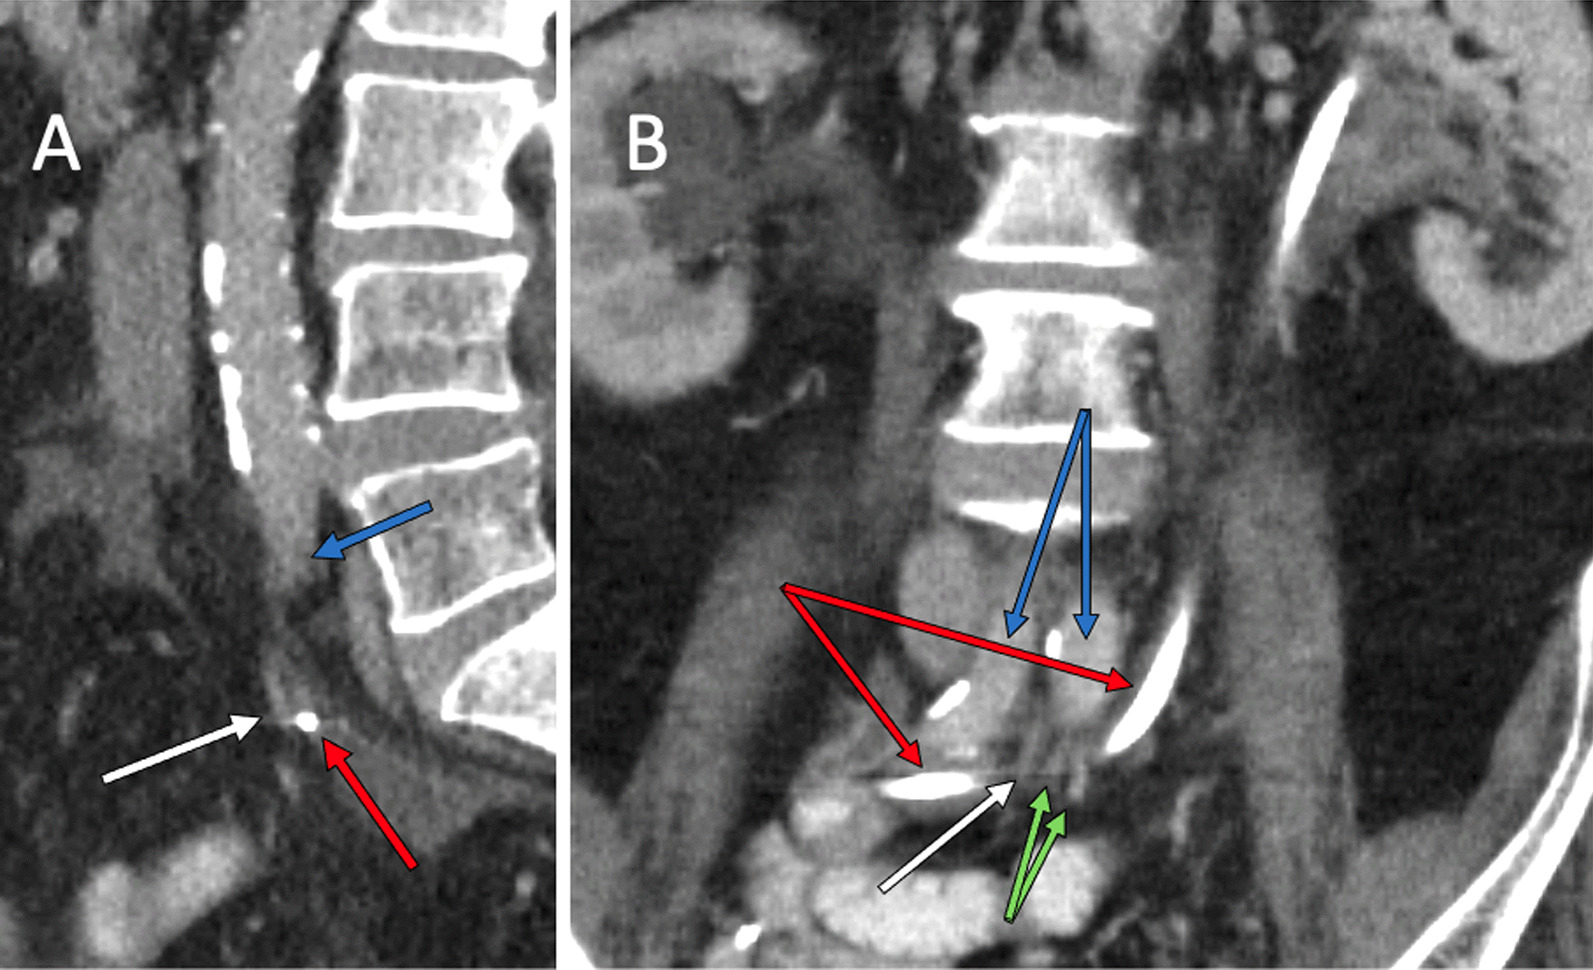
CTA of the abdomen and pelvis performed two months prior to the endovascular intervention, demonstrated intimate posterior relationship (A) of the reverse Hobbs catheter (red arrow) to three distal IMA branches (white arrow for culprit vessel and green arrows for remaining branches (A, B)), and close anterior position of Hobbs catheter to common iliac vessels (blue arrows). Note is made of bilateral hydronephrosis and left ureteropelvic blood clots
